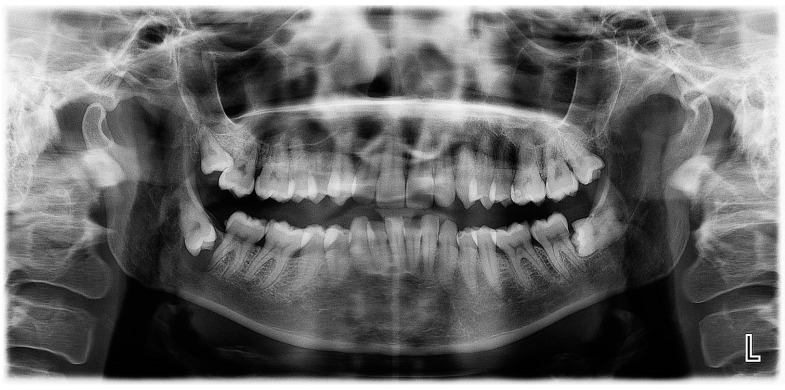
Panoramic X-ray of case #2.
